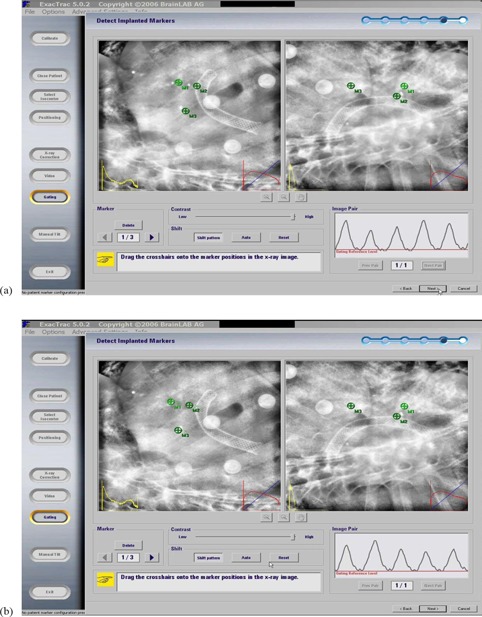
Two orthogonal X‐ray images acquired at the full exhale reference level of a liver tumor patient with the implanted fiducial markers before (a) and after (b) fusion. Markers (M1, M2, and M3) were reconstructed from planning CT of the location of the implanted fiducial markers and then were projected onto the X‐ray images.
